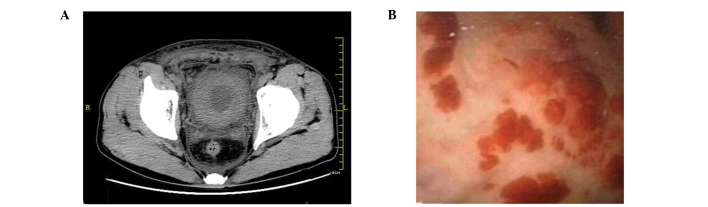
(A) Computed tomography image of the pelvis showing diffuse thickening of the bladder wall. (B) Cystoscopy observations revealed an erythematous velvety appearance of the bladder mucosa.
